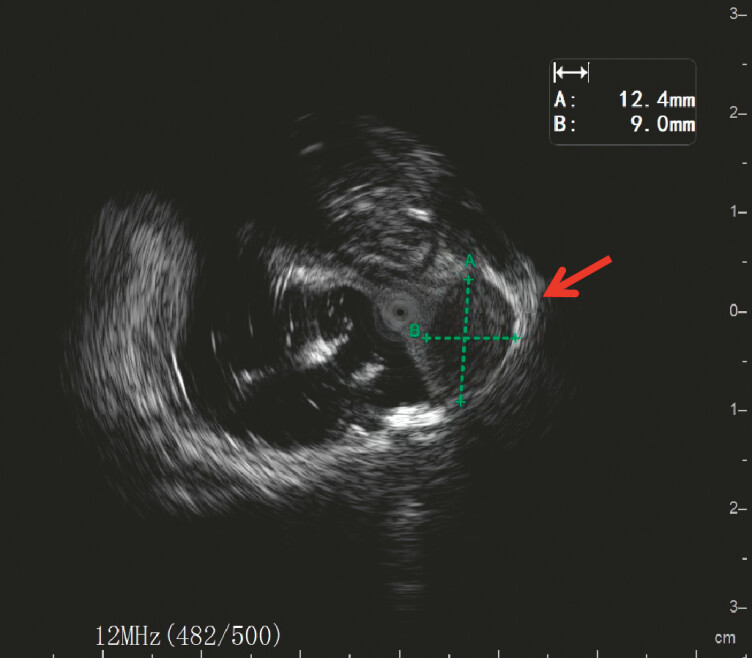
An endoscopic ultrasound image showing a hypoechoic mass within the muscularis propria of the bulb. The lesion demonstrates homogeneous echogenicity, intramural and extramural growth, and well-defined borders and measures approximately 12 × 9 mm (arrow).
